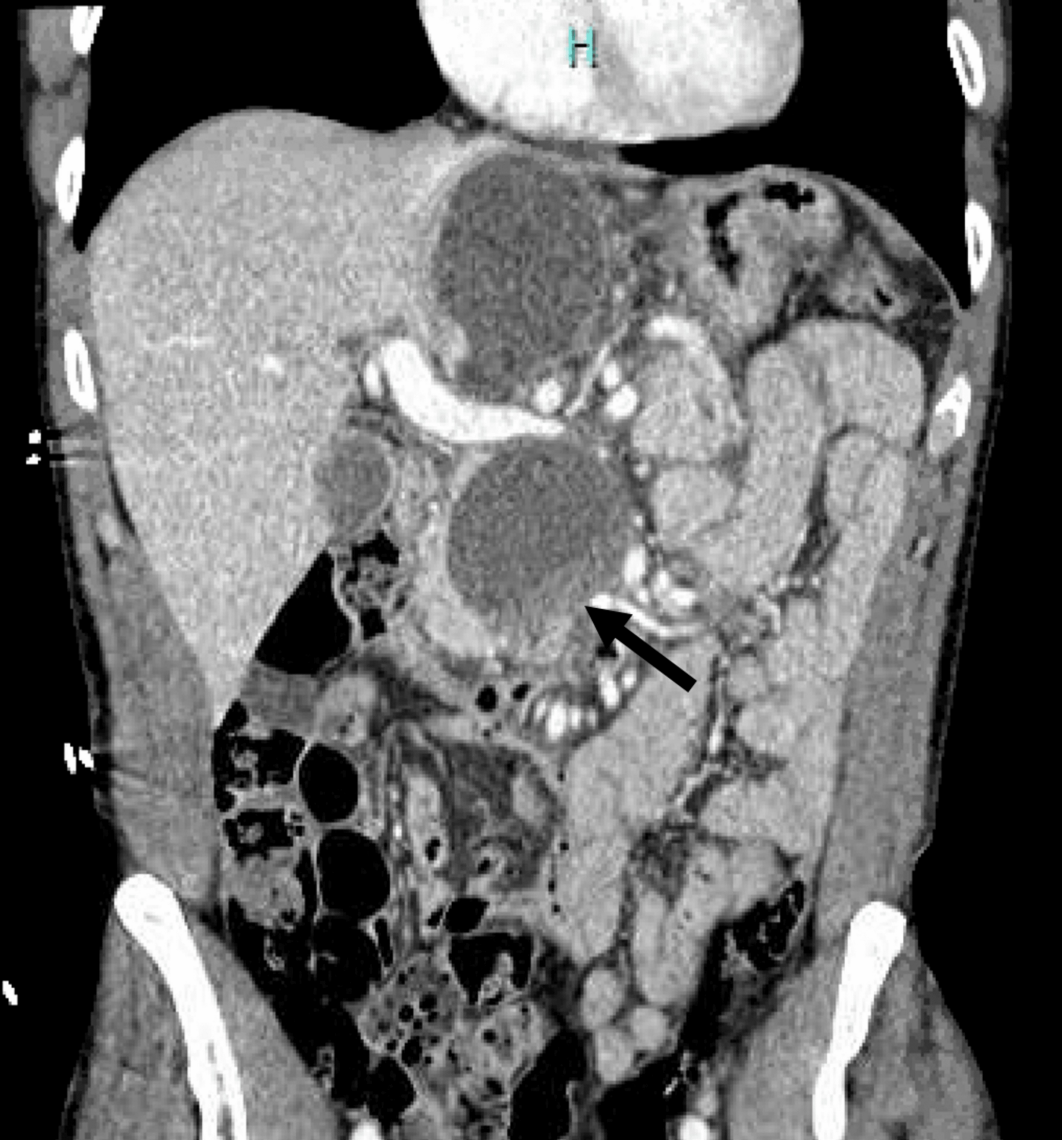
Coronal section of CT abdomen and pelvis showing a superior PPC measuring 6.6 cm, and an inferior PPC measuring 5.3 cm, with new evidence of hemorrhage (arrow) into the inferior pseudocyst CT, computed tomography; PPC, pancreatic pseudocyst
